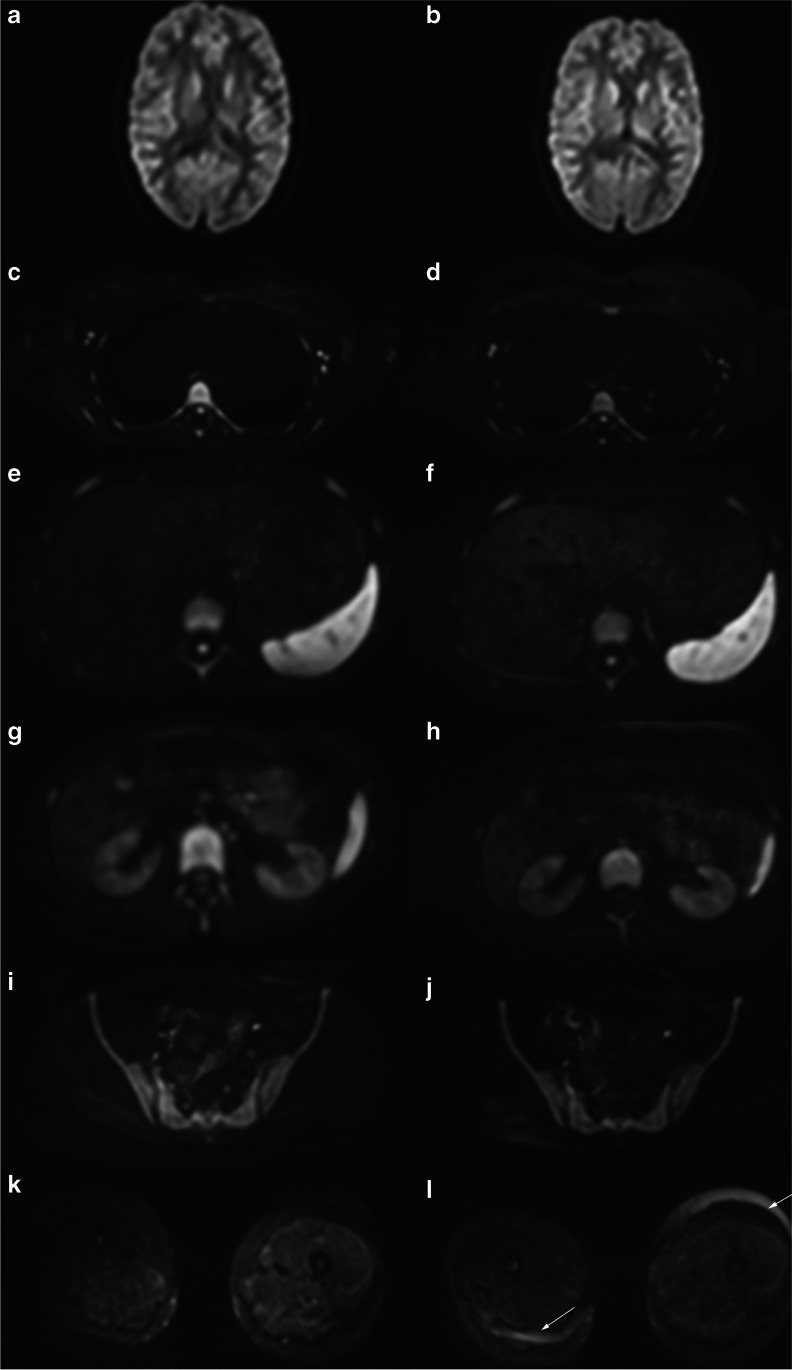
Whole-body-magnetic resonance scan in a 16-year-old girl with fever of unknown origin. Comparison of axial orientated diffusion-weighted examinations at b800 for brain (a,b), chest (c,d), upper abdomen (e,f), kidneys (g,h), pelvis (i,j) and thigh muscles (k,l). a,c,e,g,i,k Standard diffusion-weighted imaging (DWI). b,d,f,h,j,l Simultaneous multi-slice DWI. Note small field inhomogeneities of the subcutaneous fat of the limb, probably due to limited compatibility of the used coils with the simultaneous multi-slice technique (arrows in l)
